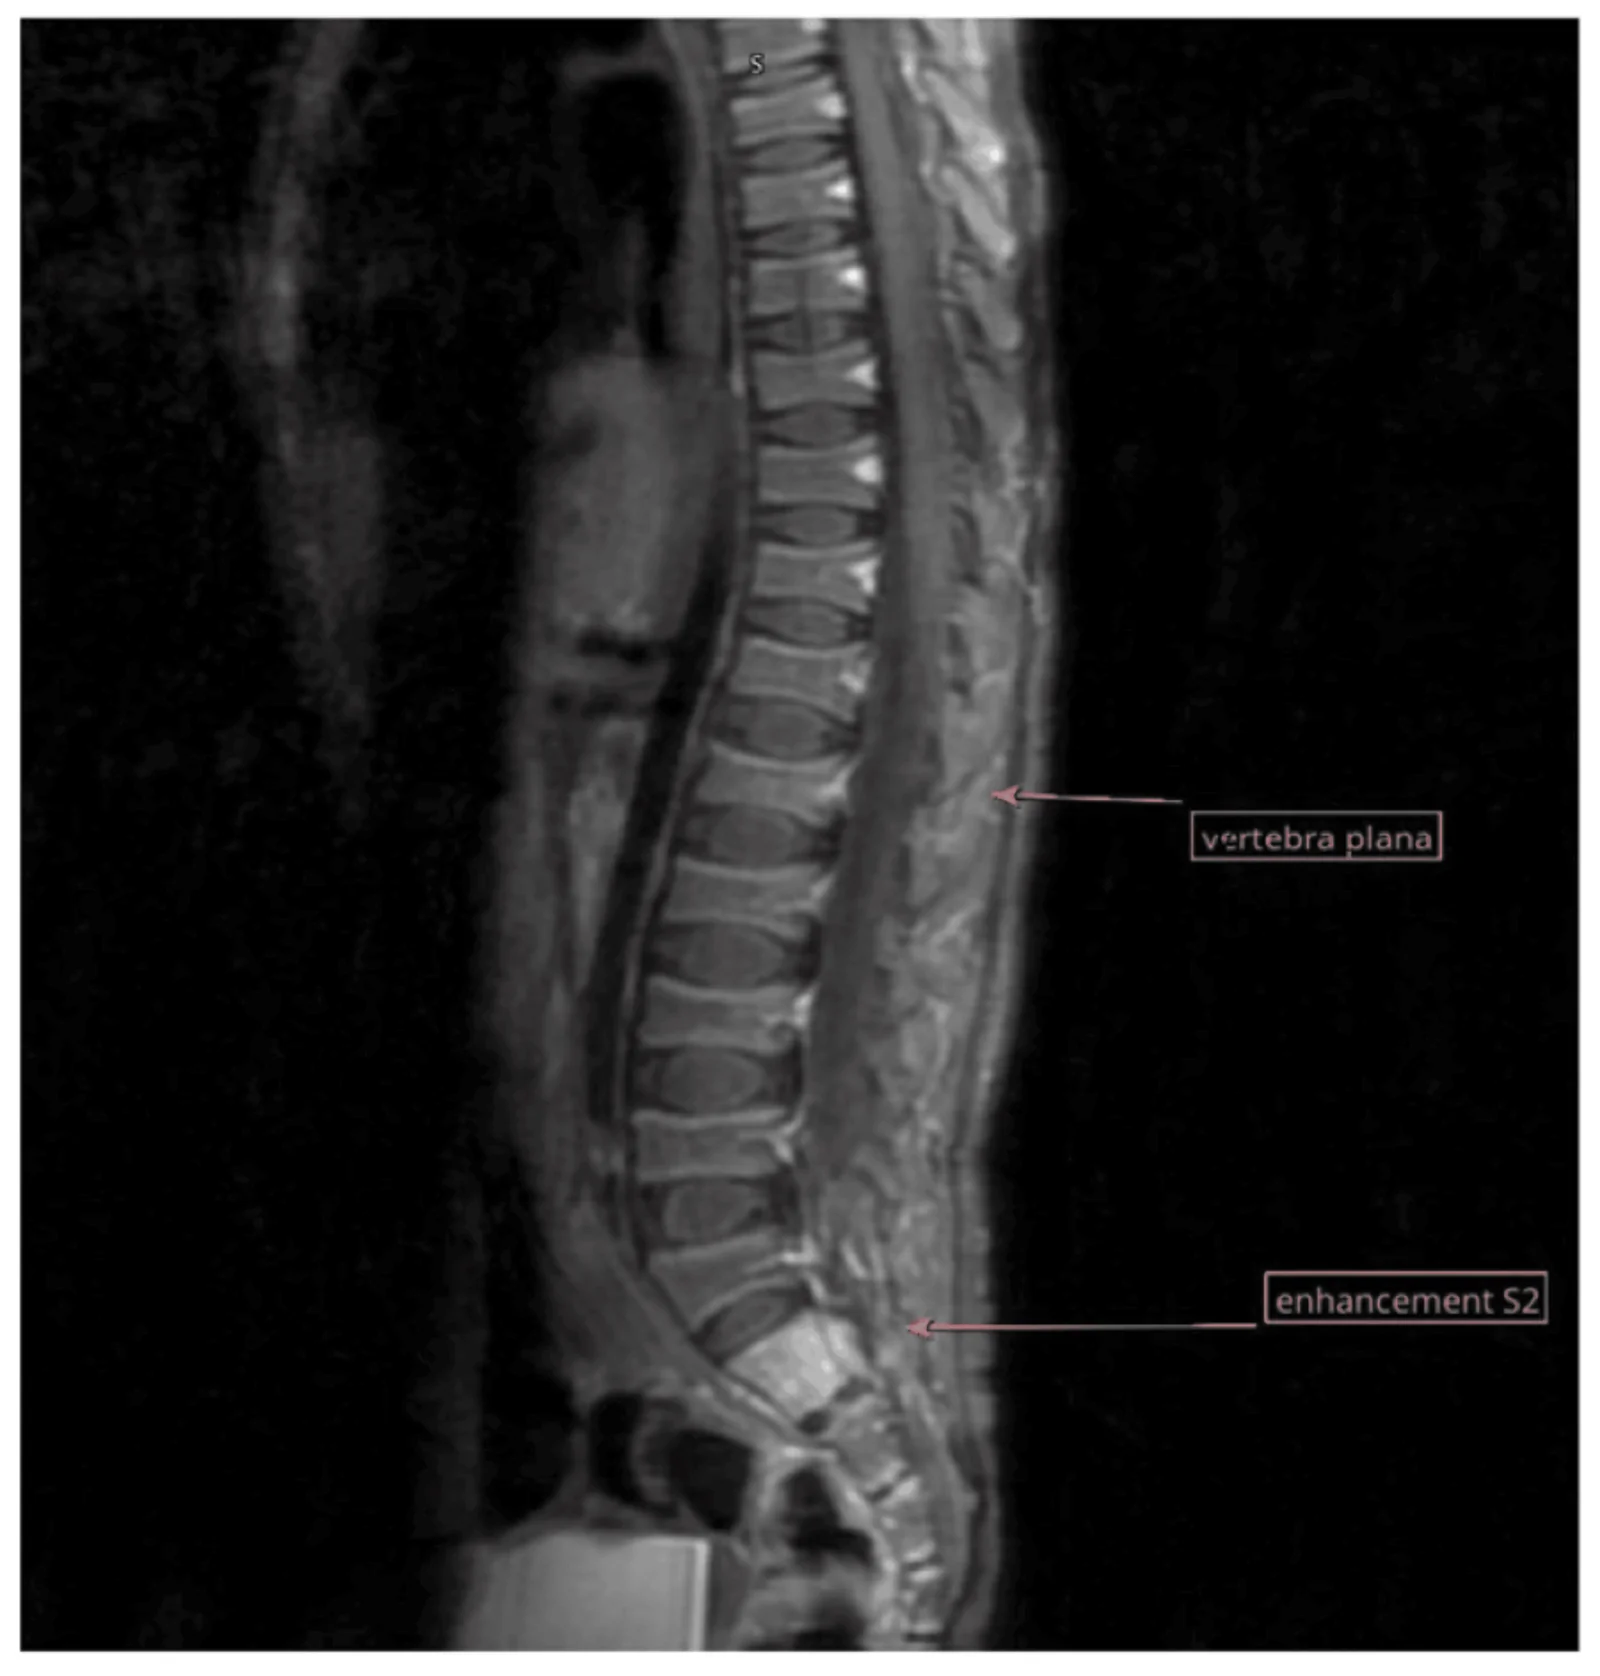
Sagittal short tau inversion recovery (STIR) MRI of the thoracolumbar spine demonstrating vertebra plana of T12 (arrow) with marrow signal abnormalities and associated sacral (S2) marrow hyperintensity, findings that contributed to the initial diagnostic consideration of chronic recurrent multifocal osteomyelitis.
